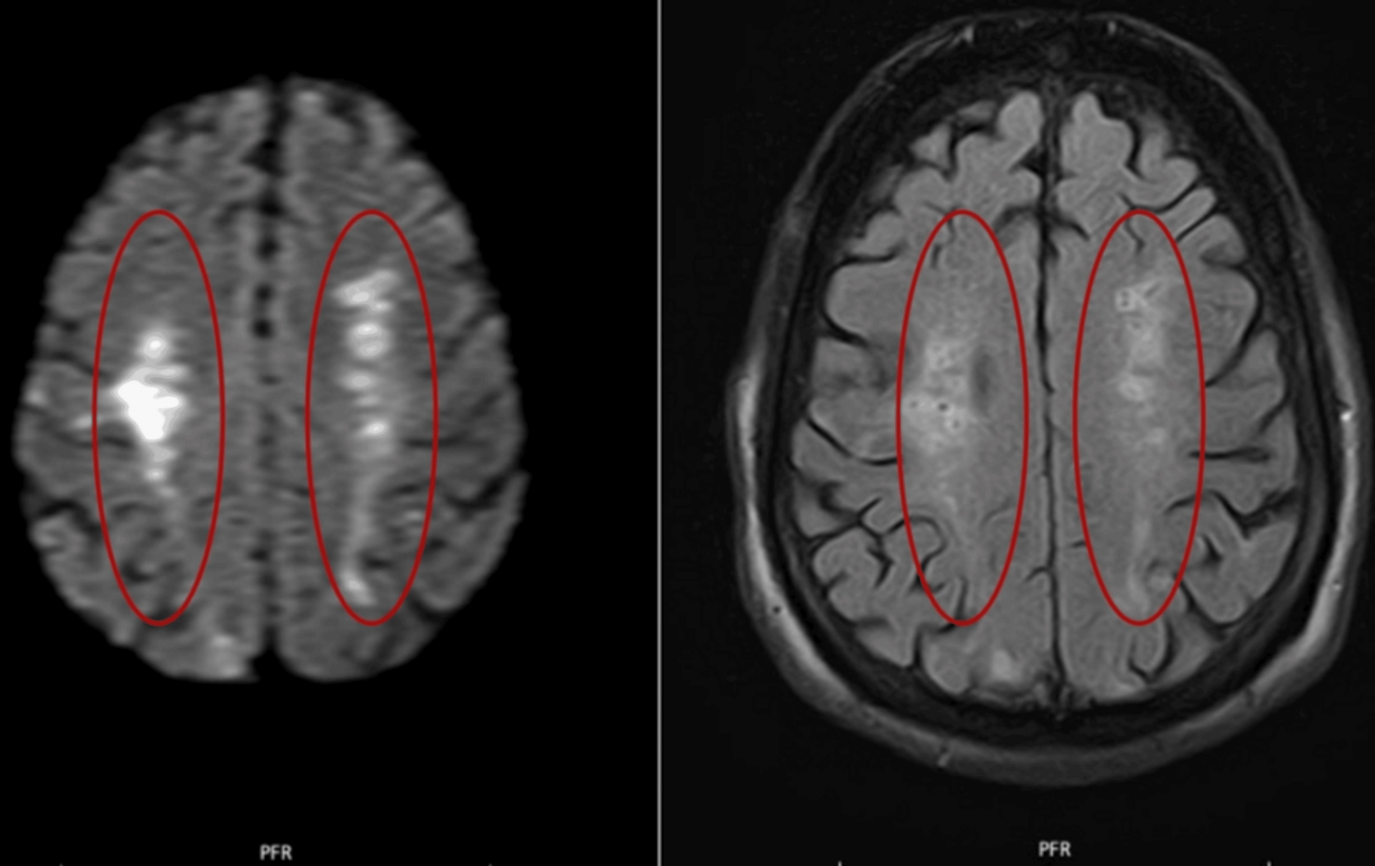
Axial DWI (left) and FLAIR (right) images – multiple recent ischemic lesions in the centrum semiovale, forming a bilateral "line" pattern, suggestive of watershed stroke. DWI: Diffusion-Weighted Imaging, FLAIR: Fluid-Attenuated Inversion Recovery
